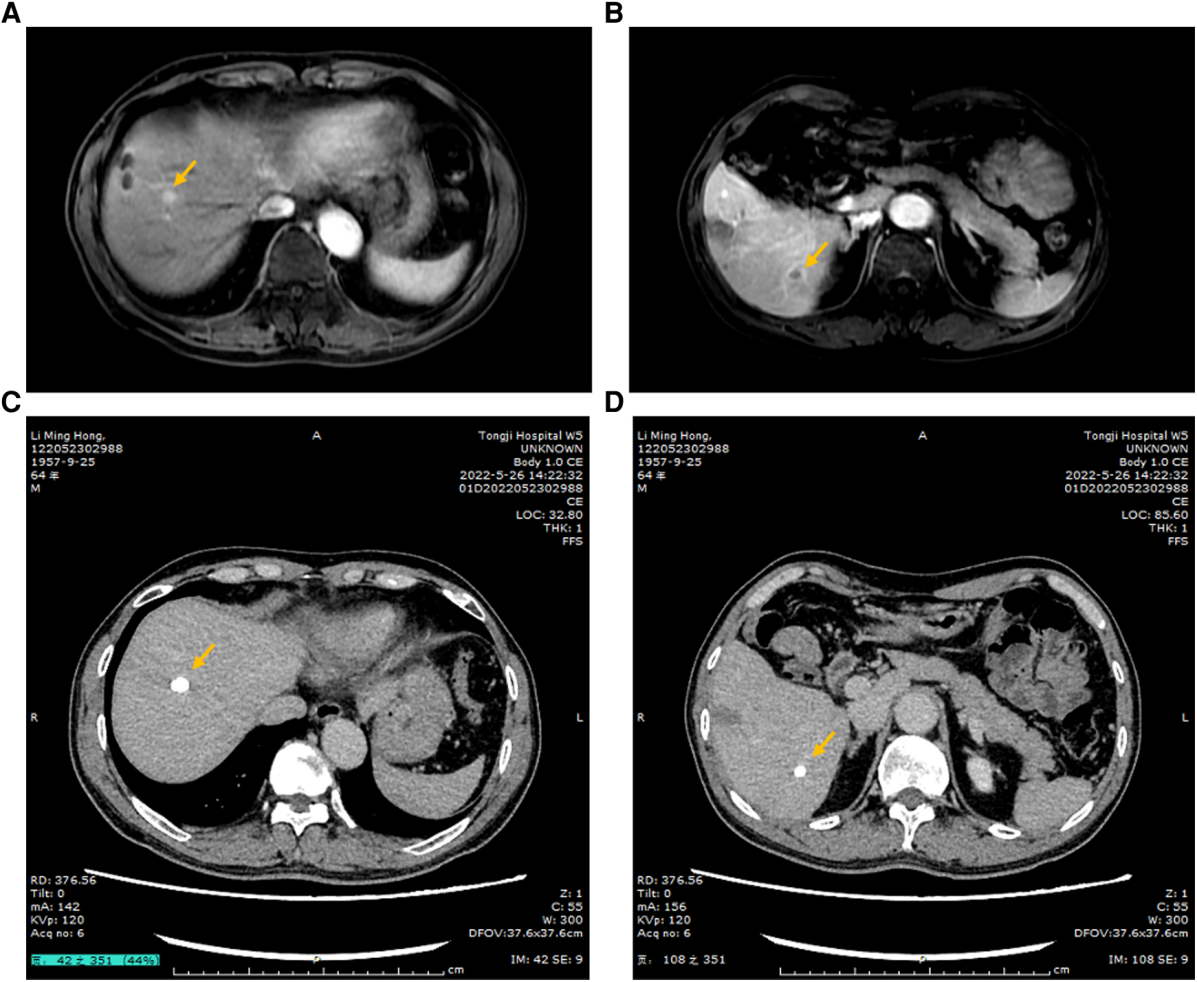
(A,B) PWI of the MRI scan showing the typical metastatic tumor in the liver; the arrow points at the lesion. (C,D) After TACE combined with angiogenesis inhibitors and ICIs, the typical lesions of the liver were covered by iodized oil, which were shown in the CT scan.
